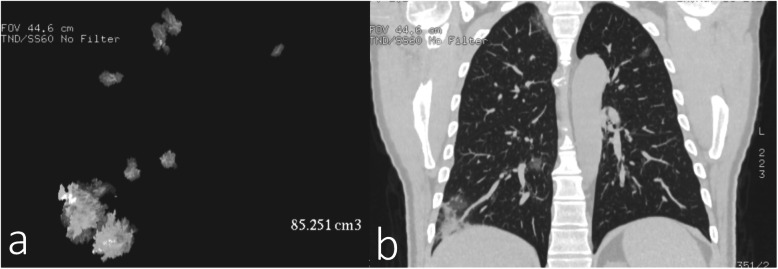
Example of severe acute respiratory syndrome coronavirus 2 lung disease segmentation. a Maximum intensity projection coronal image shows segmented lung opacities and the volume provided in cubic centimeters. b Corresponding coronal computed tomography image
